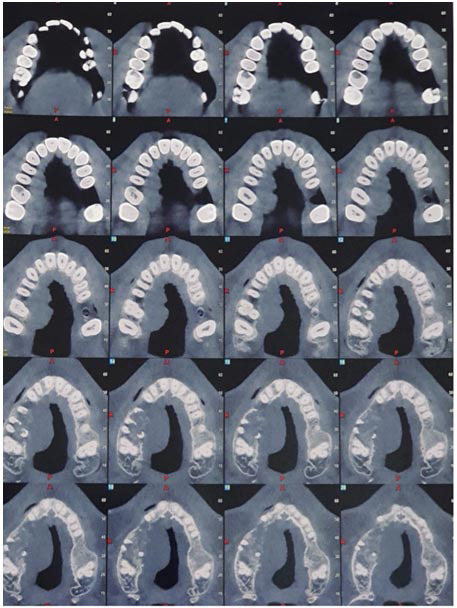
Cone beam computed tomography (CBCT) axial view showing ill-defined mixed-density lesion with a moth-eaten appearance located in the right side of the maxilla
